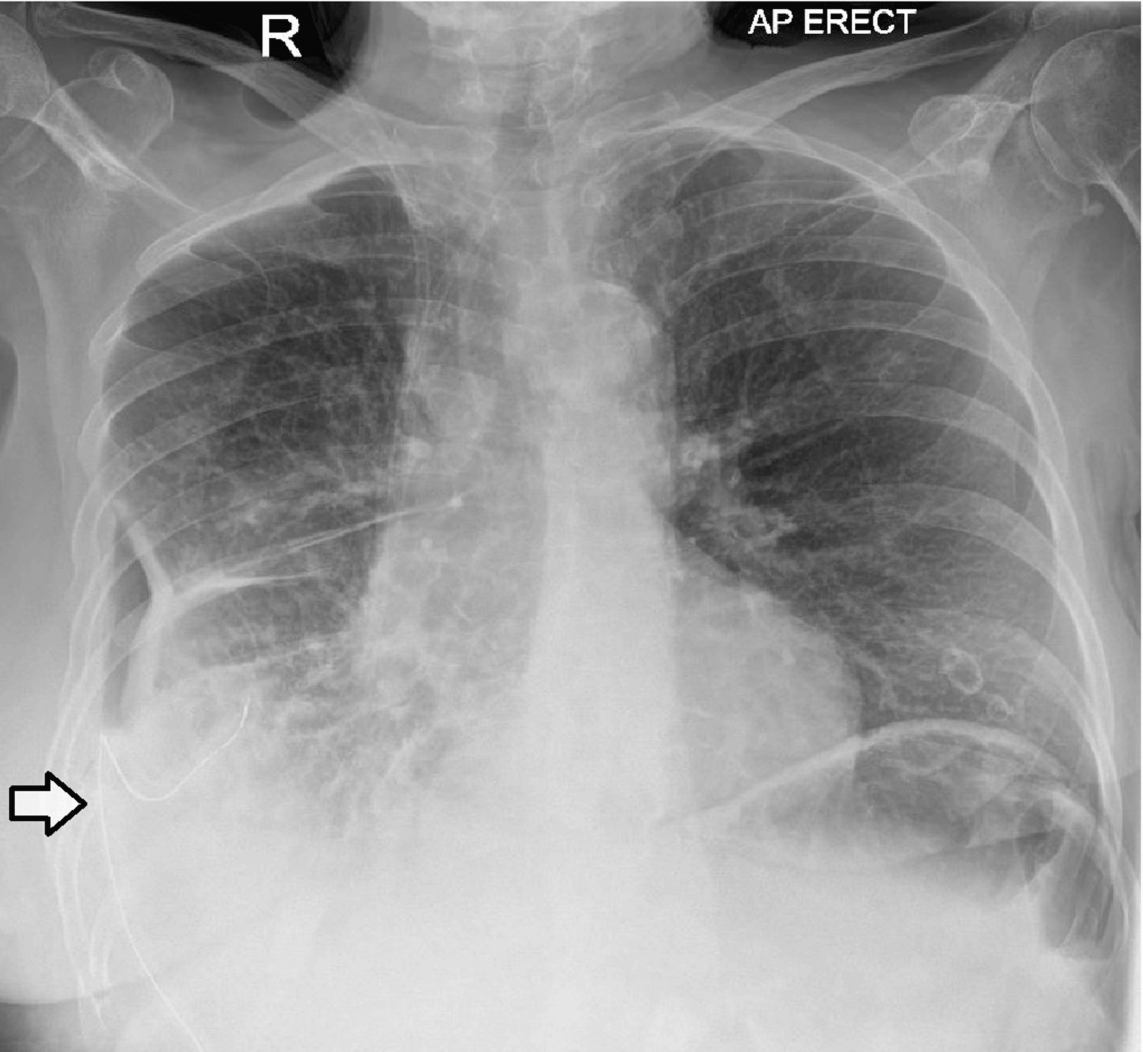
Chest X-ray following chest drain insertion
